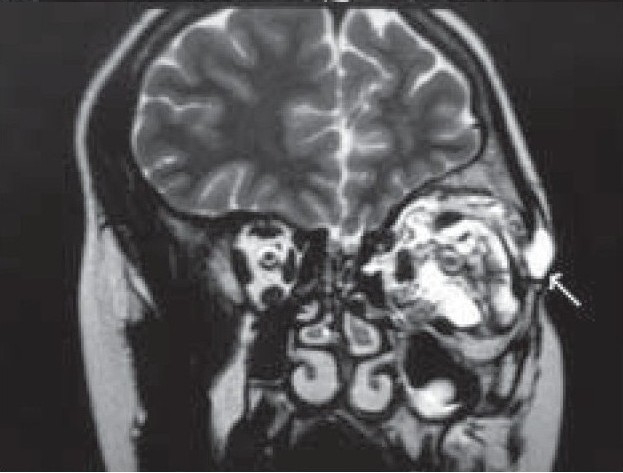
T2-coronal MRI section showing retrobulbar (intraconal) cystic spaces with extraconal extension (white arrow)
